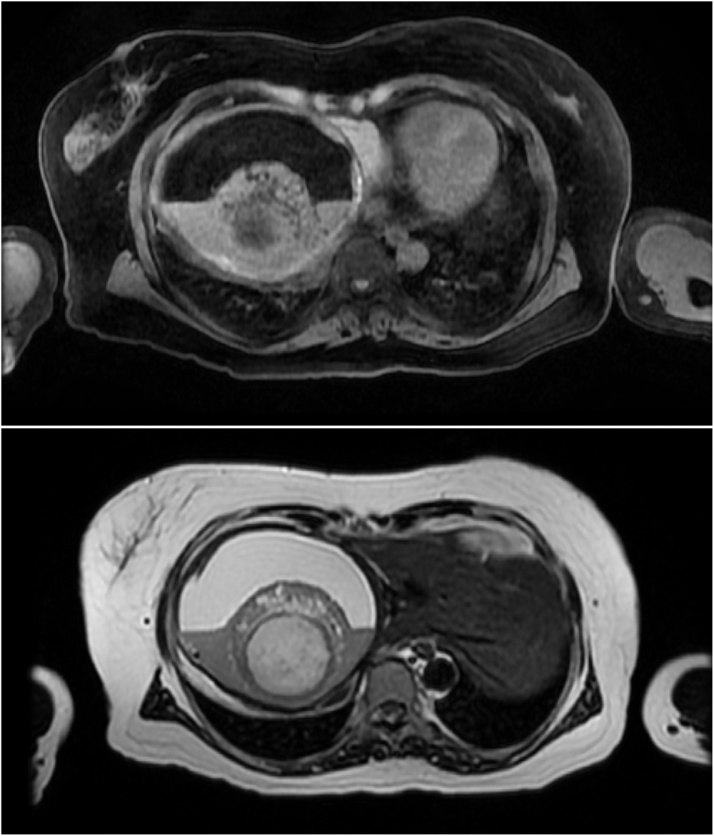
MRI Abdomen/Pelvis T1/T2 signal axial section of right upper quadrant mass with presence of fat fluid level and no internal enhancement.
